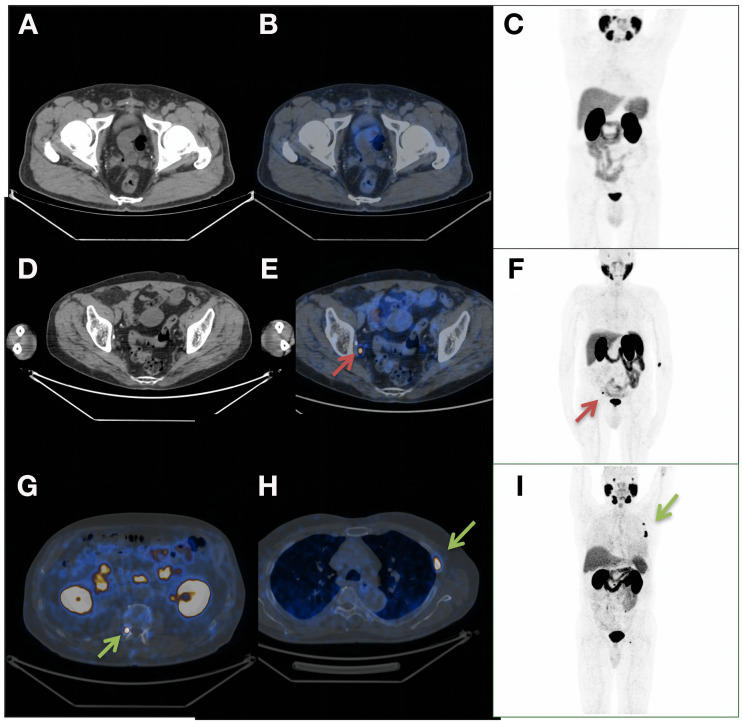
(A–C) Negative PSMA PET/CT results for 65-y-old patient who had undergone radical prostatectomy plus PNLD and had T3bN0 BCR (PSA, 0.55 ng/mL). Treatment plan was not altered by PSMA PET/CT results (radiotherapy) (A: axial CT; B: axial fusion; C: maximum-intensity projection [MIP]). (D–F) Positive PSMA PET/CT results for 67-y-old patient who had undergone radical prostatectomy plus PNLD and who had T2aN1 BCR (PSA, 0.4 ng/mL). Treatment plan was modified from radiotherapy to ADT (D: axial CT; E: axial fusion; F: MIP) for 0.4-cm lymph nodes (red arrows). (G–I) Positive PSMA PET/CT results for 65-y-old patient who had undergone radical prostatectomy plus PNLD and who had T3aN0 BCR (PSA, 0.2 ng/mL). Treatment plan was modified from radiotherapy to chemotherapy (G: axial CT; H: axial fusion; I: MIP) for metastatic bone lesions (green arrows). PNLD = pelvic lymph node dissection.
